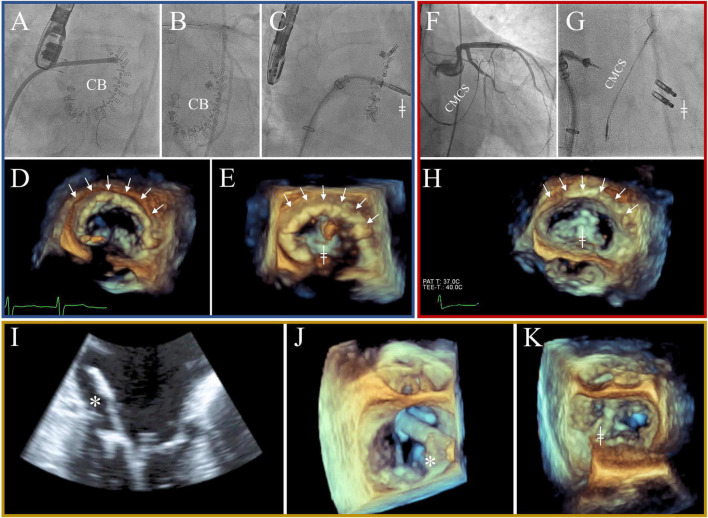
All three combination (COMBO) strategies with blue: Cardioband + mitral transcatheter edge-to-edge repair (M-TEER) (A–E), red: Carillon Mitral Contour System (CMCS) + M-TEER (F–H), and orange: Neochord + M-TEER (I–K). Blue: Cardioband is implanted using fluoroscopy [A + B; “Cardioband (CB)” and arrows] and 3D echocardiography (D). Intermittently, unimpaired flow of left circumflex coronary artery (LCX) is checked (B). Red: CMCS is implanted using fluoroscopy (F + G). Intermittently, unimpaired flow of LCX is checked (F). Note the pull of the CMCS in the left atrium displayed in 3D echocardiography (arrows). Orange: Neochord is implanted using echocardiography [2D: (I) and 3D: (J)]. Please note the open jaws of the NeoChord Implanting system, ready to grasp the flailing posterrior mitral leaflet (*). MC is implanted second to either Cardioband (C,E), CMCS (G,H), or Neochord (K), using both fluoroscopy, and 3D echocardiography (╪).
